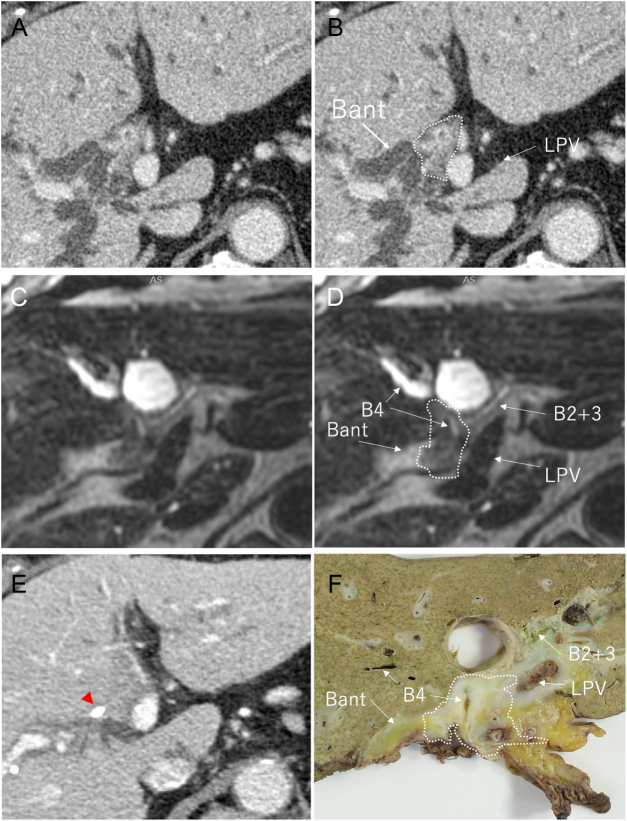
Radiological and pathological findings of a representative patient with Bismuth type IV PHC. Broken line, tumor; red triangle, EBS. (A, B) MDCT without EBS. (C, D) Cube + EBS. (E) MDCT + EBS, (F) Macroscopic findings. Broken line, tumor annotation based on the pathological finding. Bant, right anterior bile duct; B4, bile duct of segment 4; EBS, endoscopic biliary stent; LPV, left portal vein; MDCT, multidetector computed tomography; PHC, perihilar cholangiocarcinoma.
